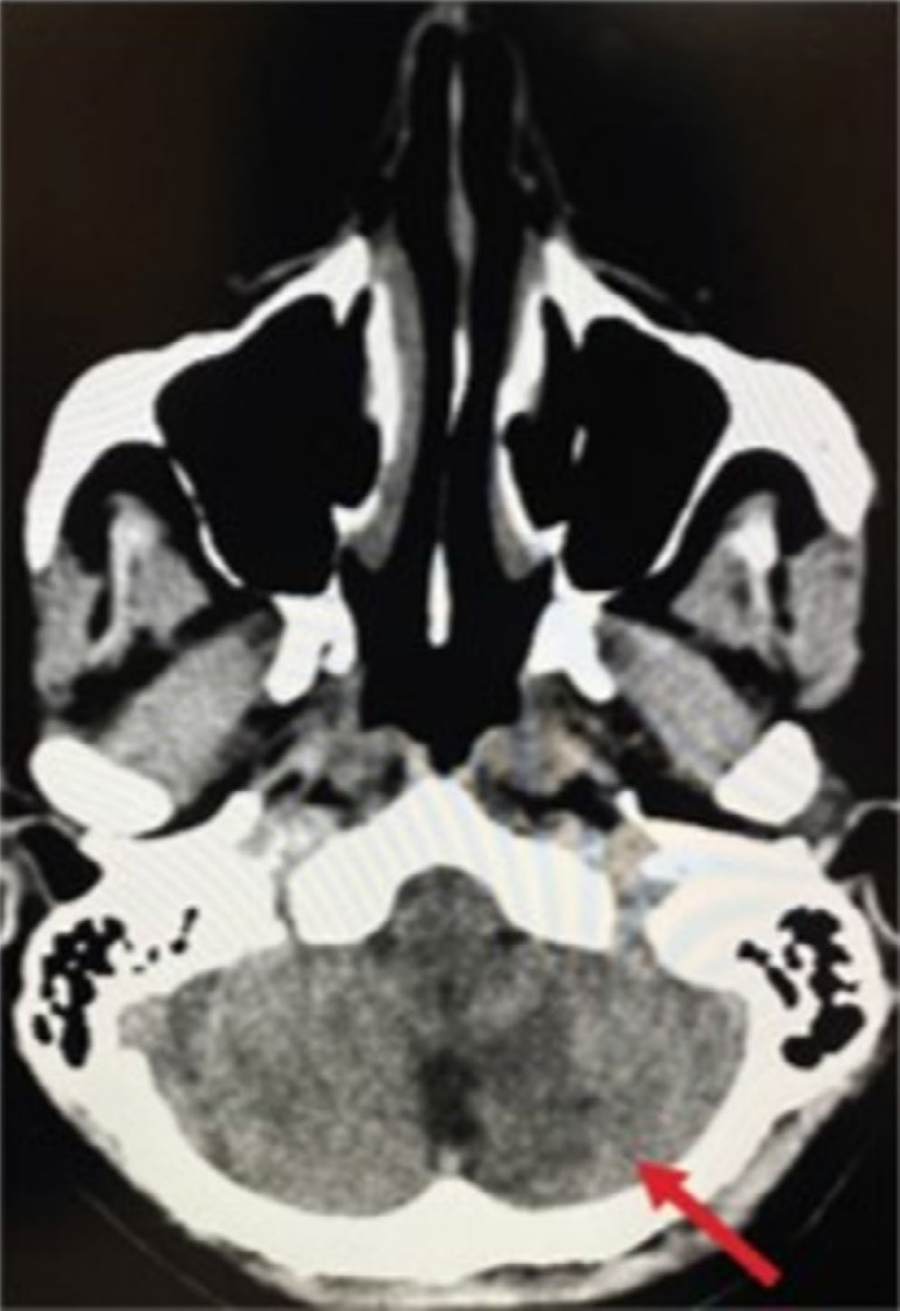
Shows a brain CT scan. The arrow points to subtle hypodensities in the left cerebellar hemisphere. These hypodensities may indicate various underlying conditions such as ischemia, infarction, or other pathological changes.
